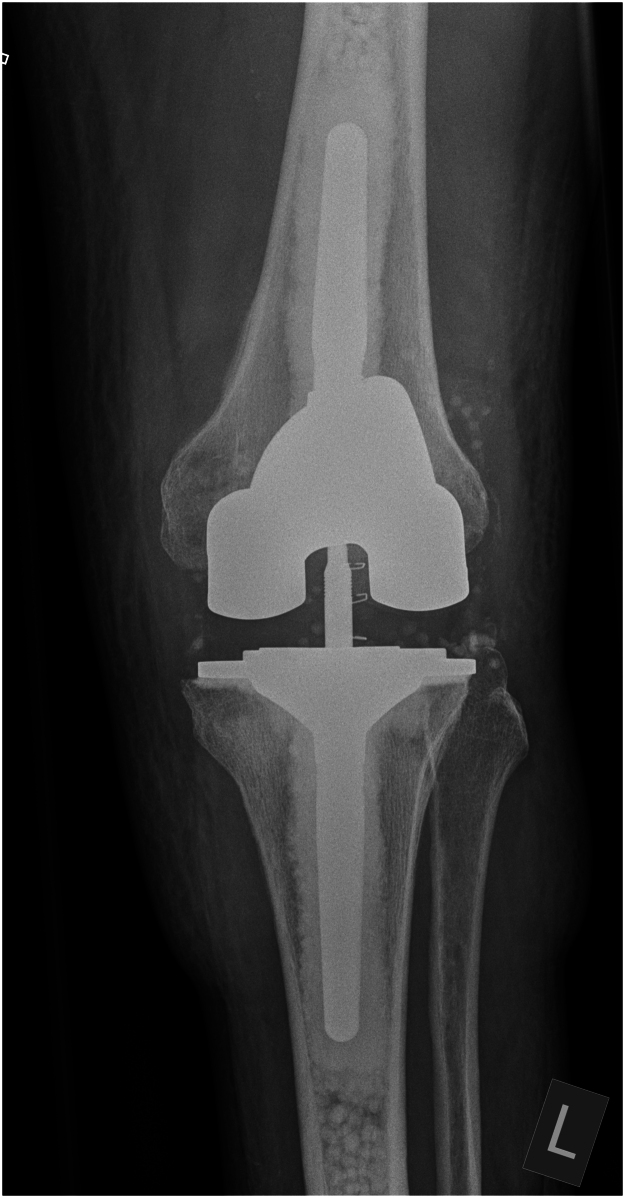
Revision total knee replacement immediately post surgery. The anteroposterior x-ray of the knee demonstrates uniformly distributed antibiotic-loaded calcium sulfate beads in the medullary canal. The beads are visible as small, discrete radio-opaque spheres in the distal femur and proximal tibia, proximal to the implant and cement mantle, indicating effective cement restriction.
